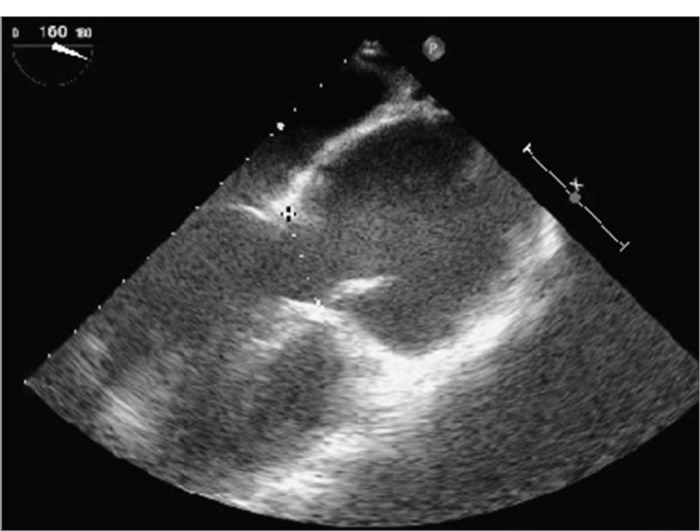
Echocardiography showing aortic root dilation to 67 mm in diameter on a long-axis view, in a 34-year-old male patient with Marfan's syndrome.
